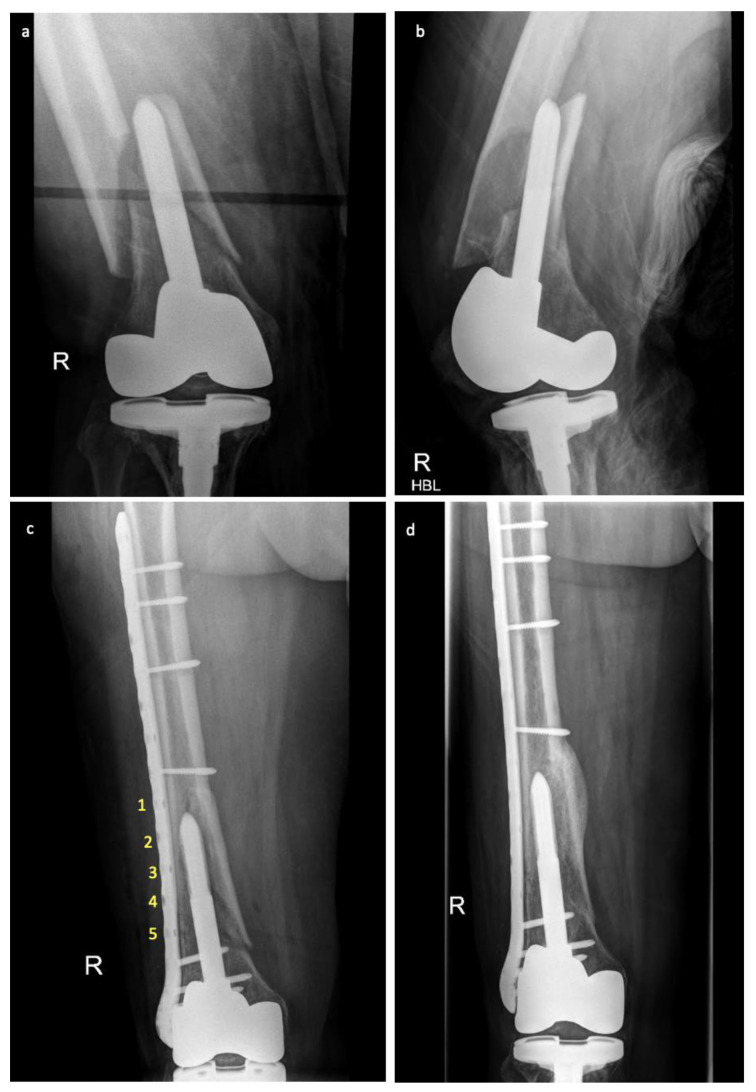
Plain film radiographs pre- and post-fracture fixation with far cortical locking screws for a periprosthetic distal femur fracture. (a) Anterior-posterior and (b) lateral views of the initial fracture. (c) Anterior-posterior view (working length labelled 1–5) day one and (d) six months post-fracture fixation with far cortical locking screws and plate constructs showing evidence of callus formation.
